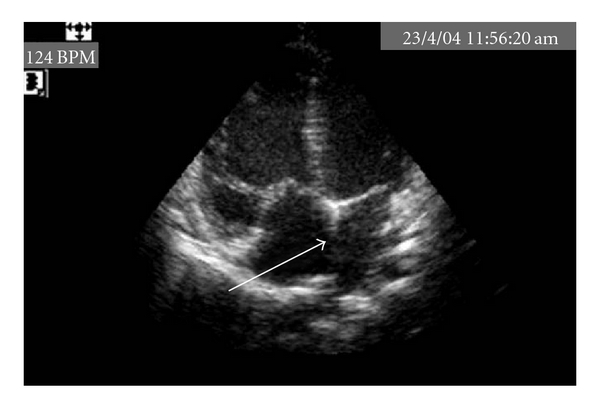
Echocardiography showing ostium secundum atrial septal defect.
